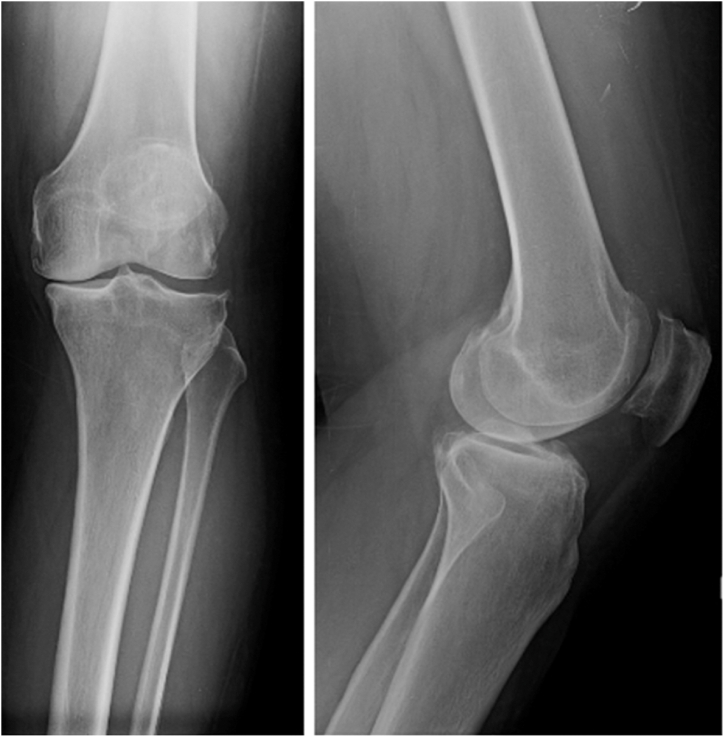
Anterior-posterior and lateral X-rays of the left knee prior to initial UKA.
